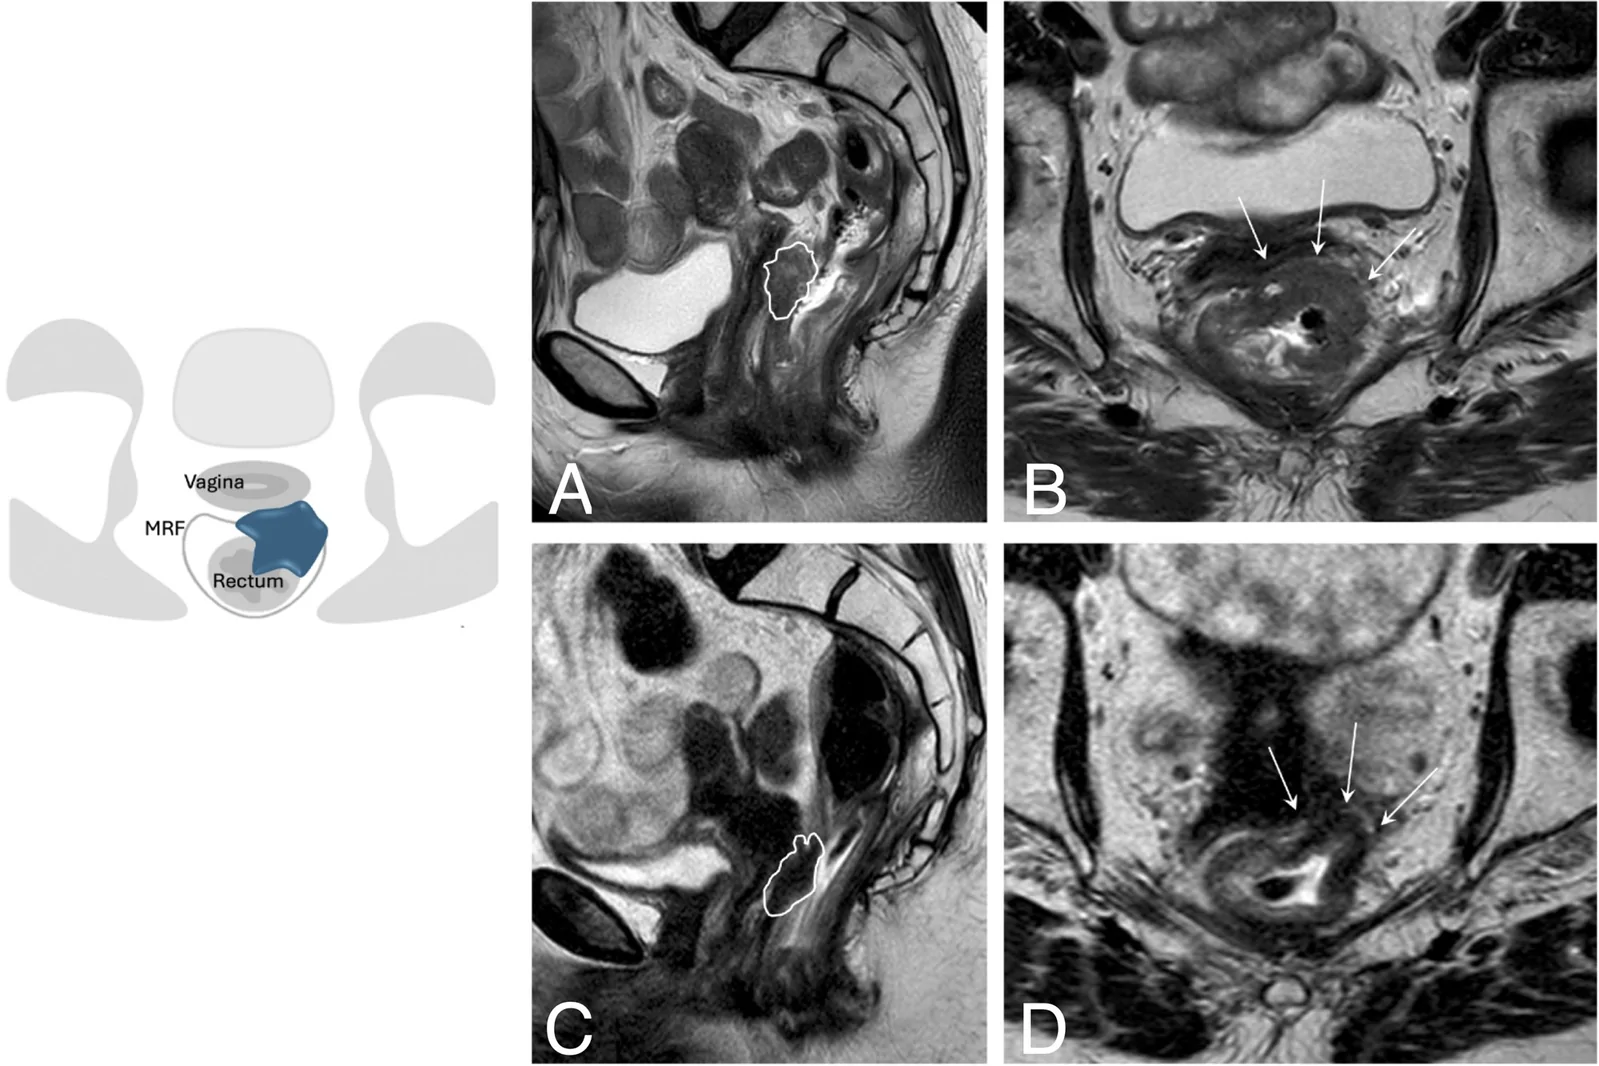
Female patient with an anastomotic recurrence following TME (low anterior resection). Baseline sagittal (A) and axial oblique (B) T2-weighted images show broad contact with the posterior vaginal wall (arrows). After chemoradiotherapy (C, D), fibrosis persists with no clear fat plane. Pathology confirmed vaginal wall invasion. Key point: loss of fat planes due to fibrosis after treatment does not indicate clear surgical margins.
